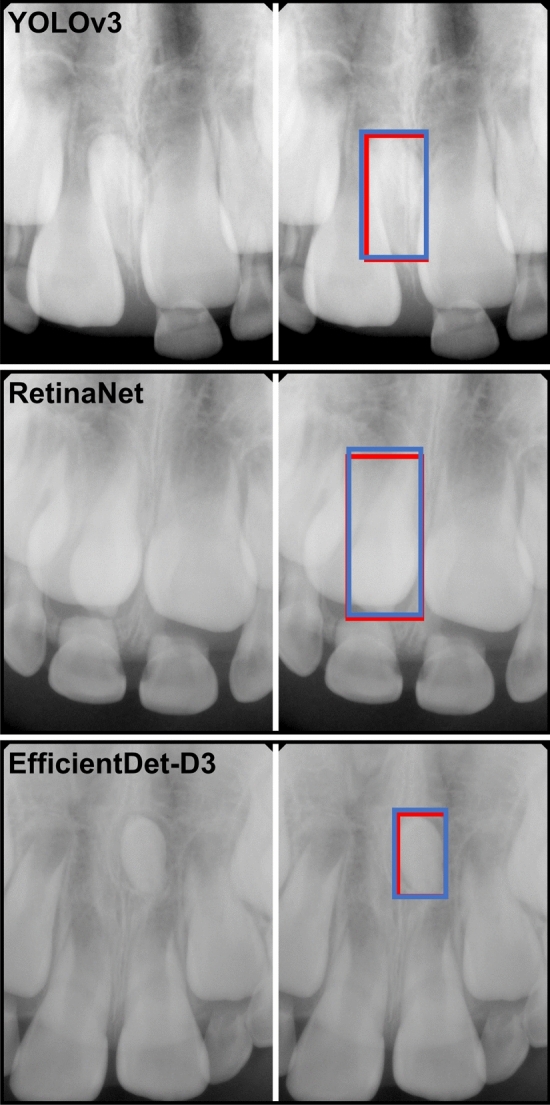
Examples of correctly detected mesiodens in the YOLOv3, RetinaNet, and EfficientDet-D3 models. The left side of each case is the original periapical radiograph, and the right side is the result of each model. The manually annotated label by the radiologist is shown as the blue box and the automatically detected mesiodens is shown as the red box.
